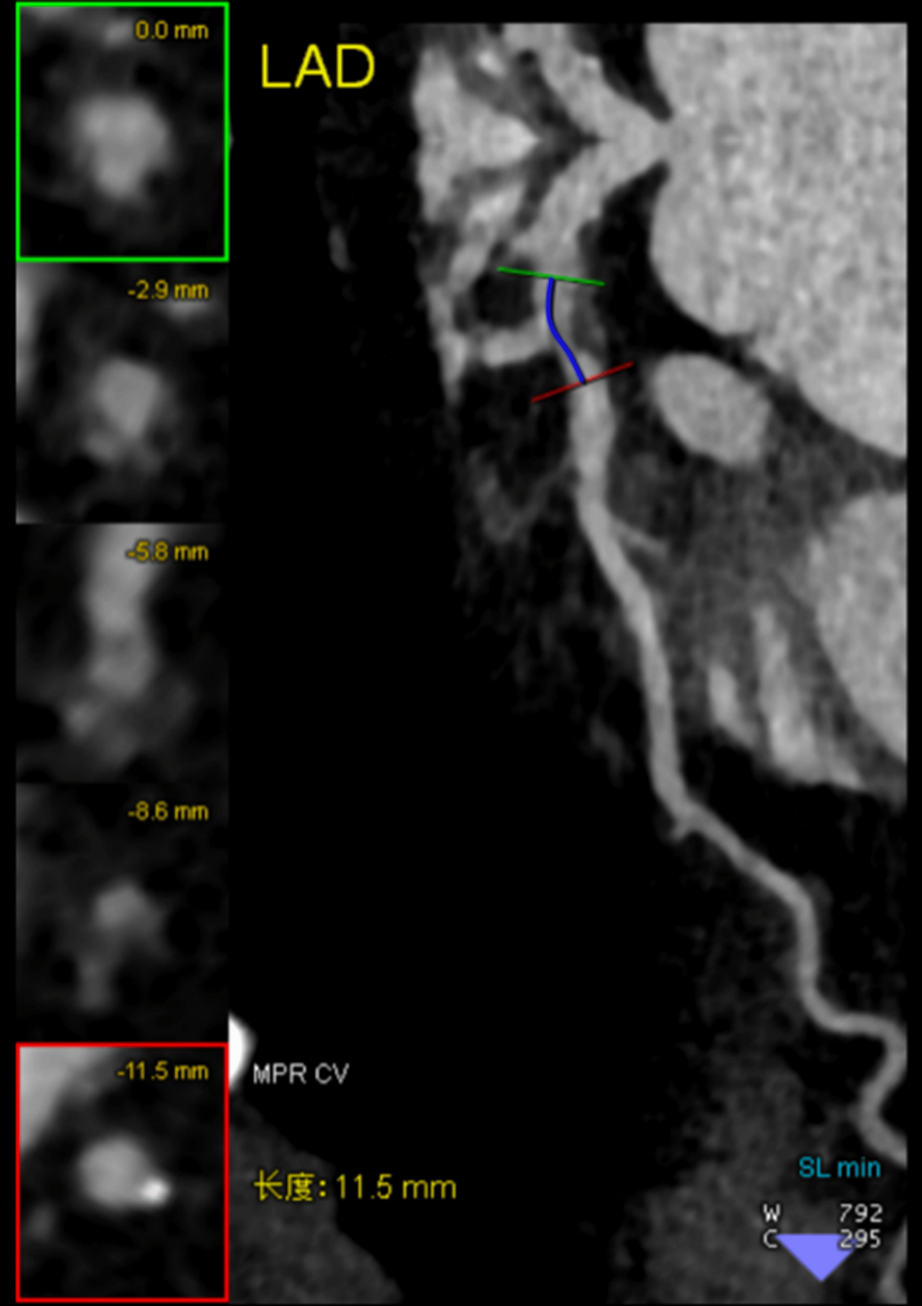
Stenosis (11.5 mm in length) of the left anterior descending artery (LAD) as seen on coronary computed tomography angiography.
